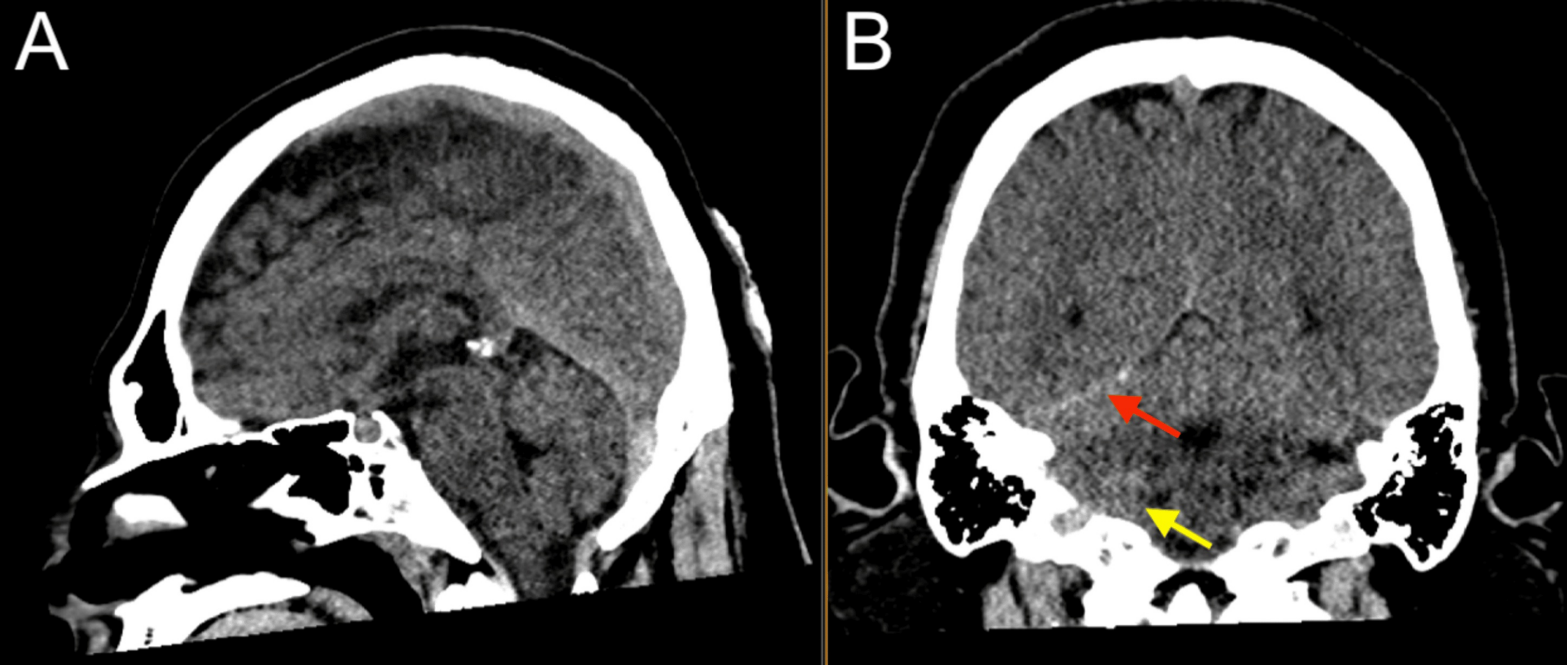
Head CT showing trace right posterior fossa and tentorial leaflet subdural hemorrhage (red), and trace right cerebellar subarachnoid hemorrhage (yellow). (A) Sagittal view; (B) Coronal view CT: computed tomography
